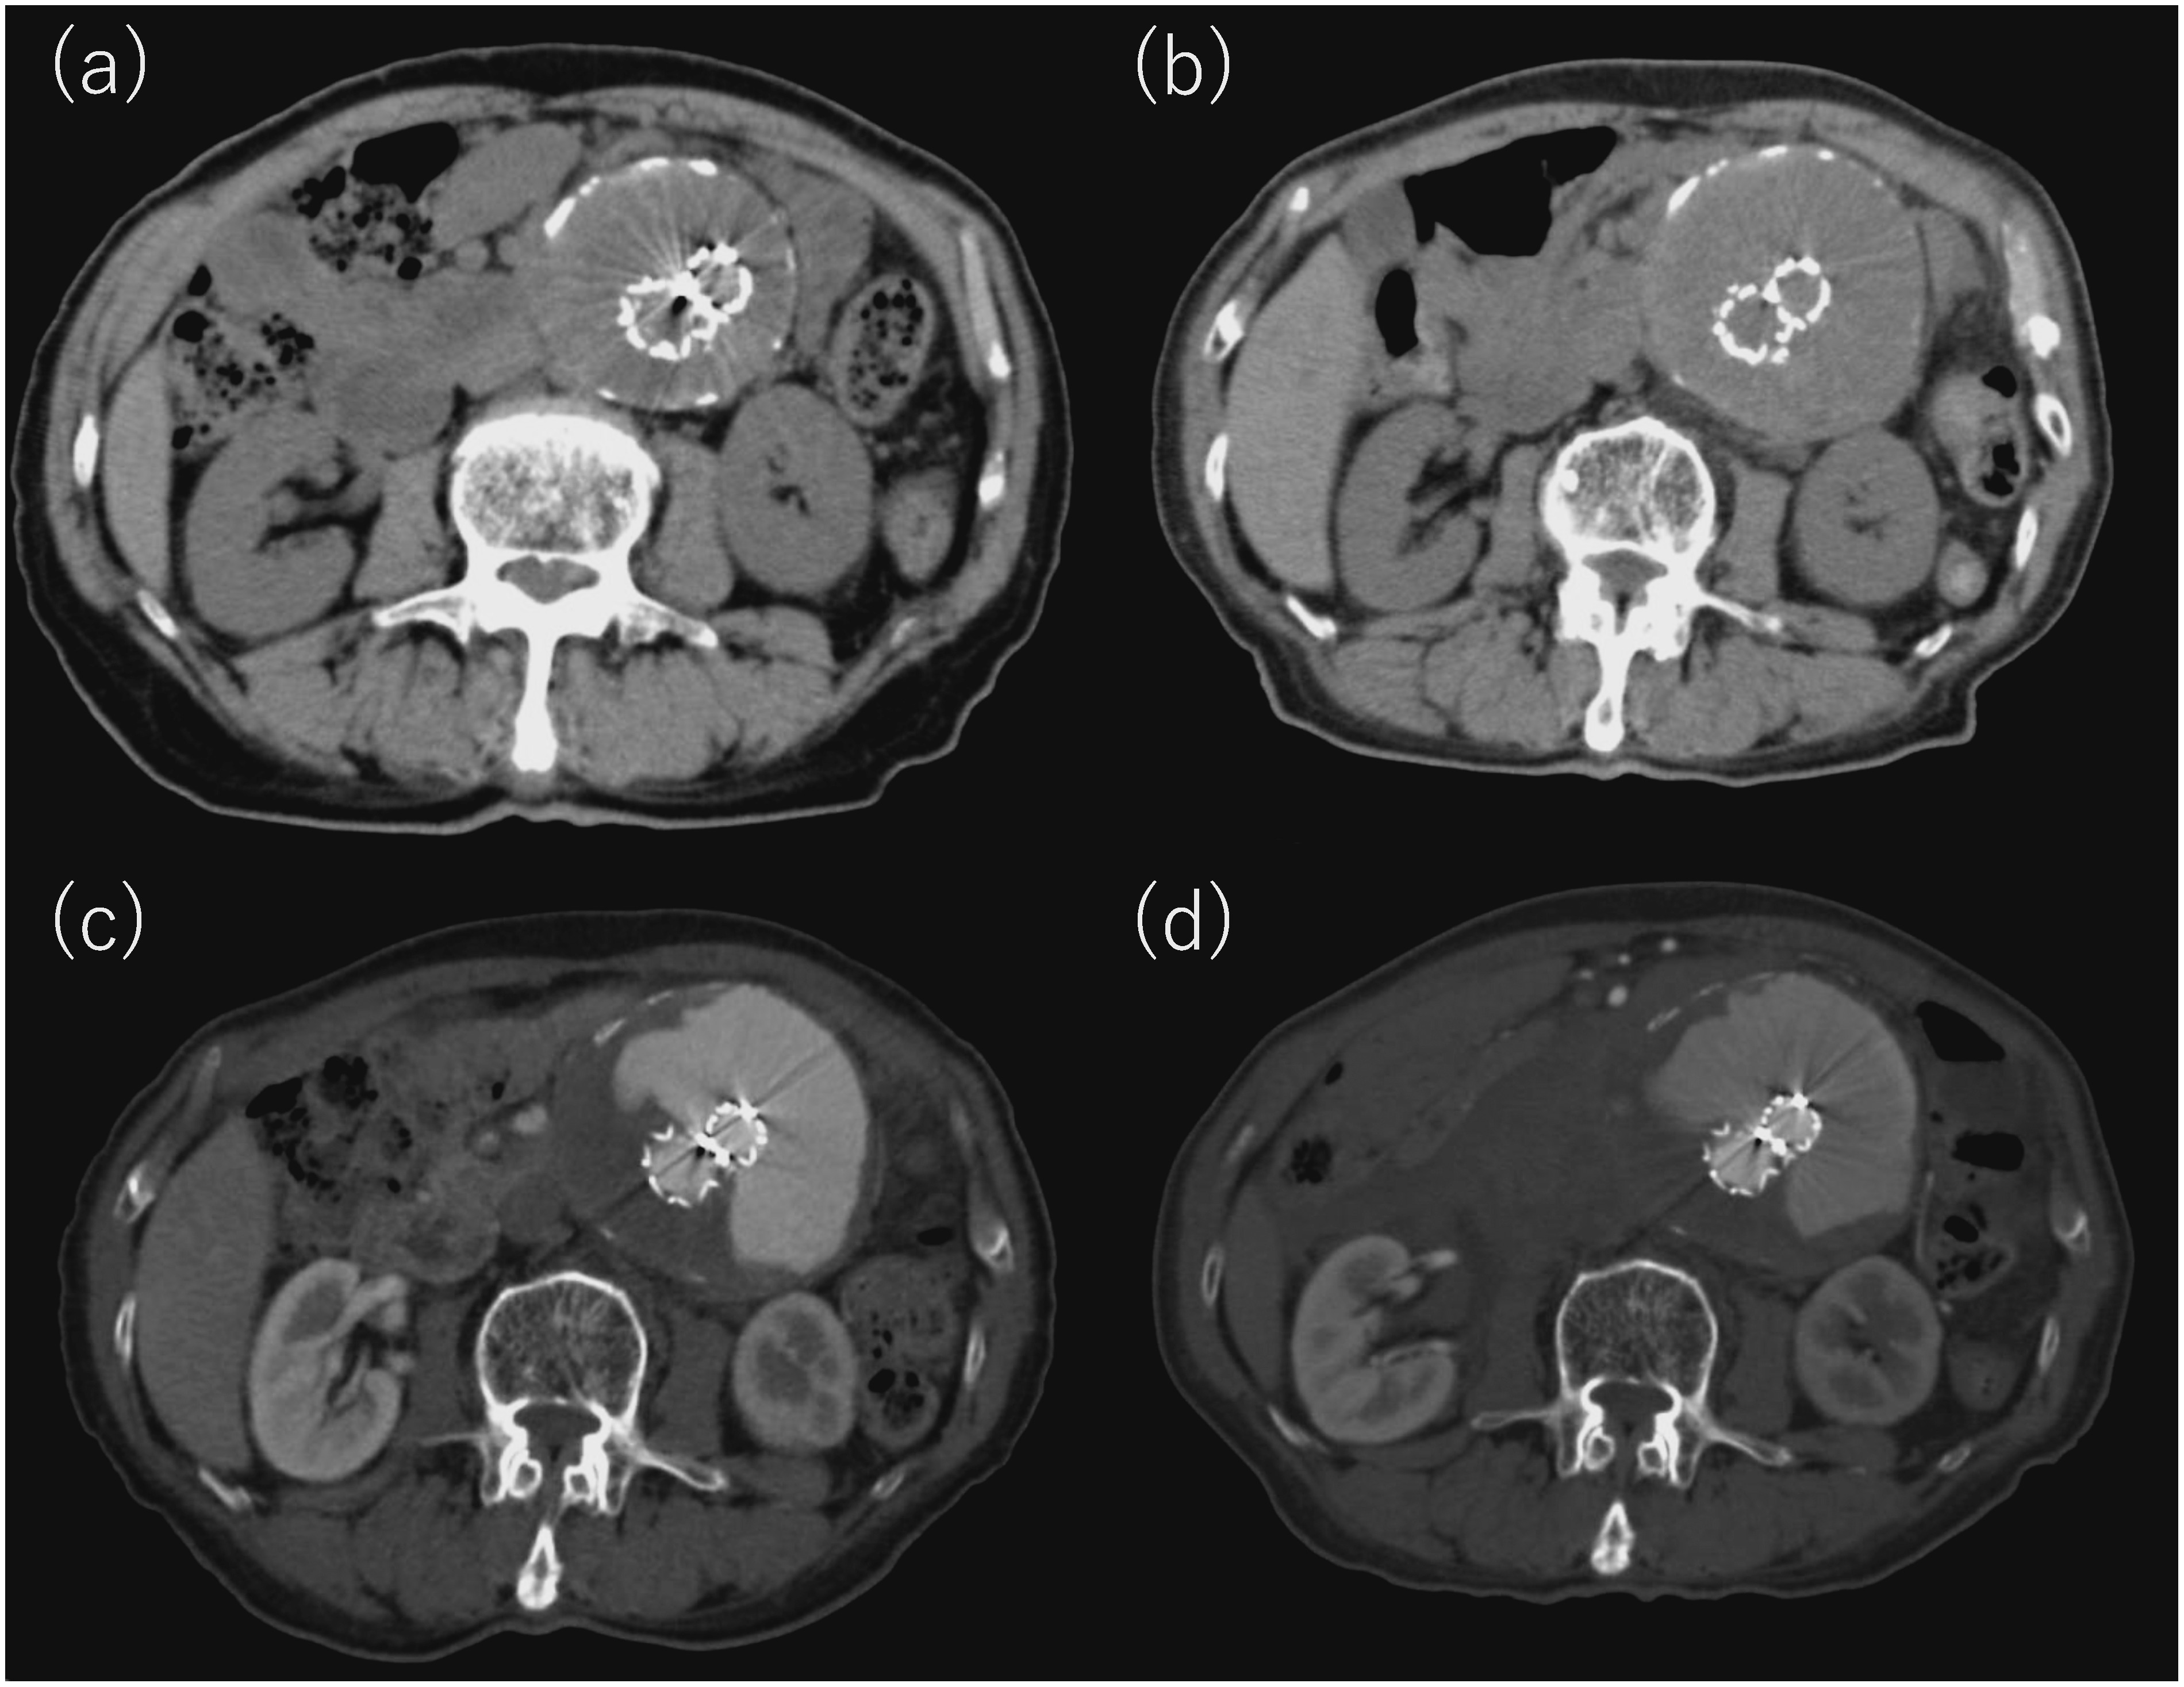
Fig. 1 Abdominal computed tomographic scan demonstrated. (a) No progressive dilatation of the aneurysm with its diameter of 60 mm, (b and c) aneurysmal dilatation of up to 75 mm in diameter and endoleak around the left leg, (d) aneurysm rupture.
